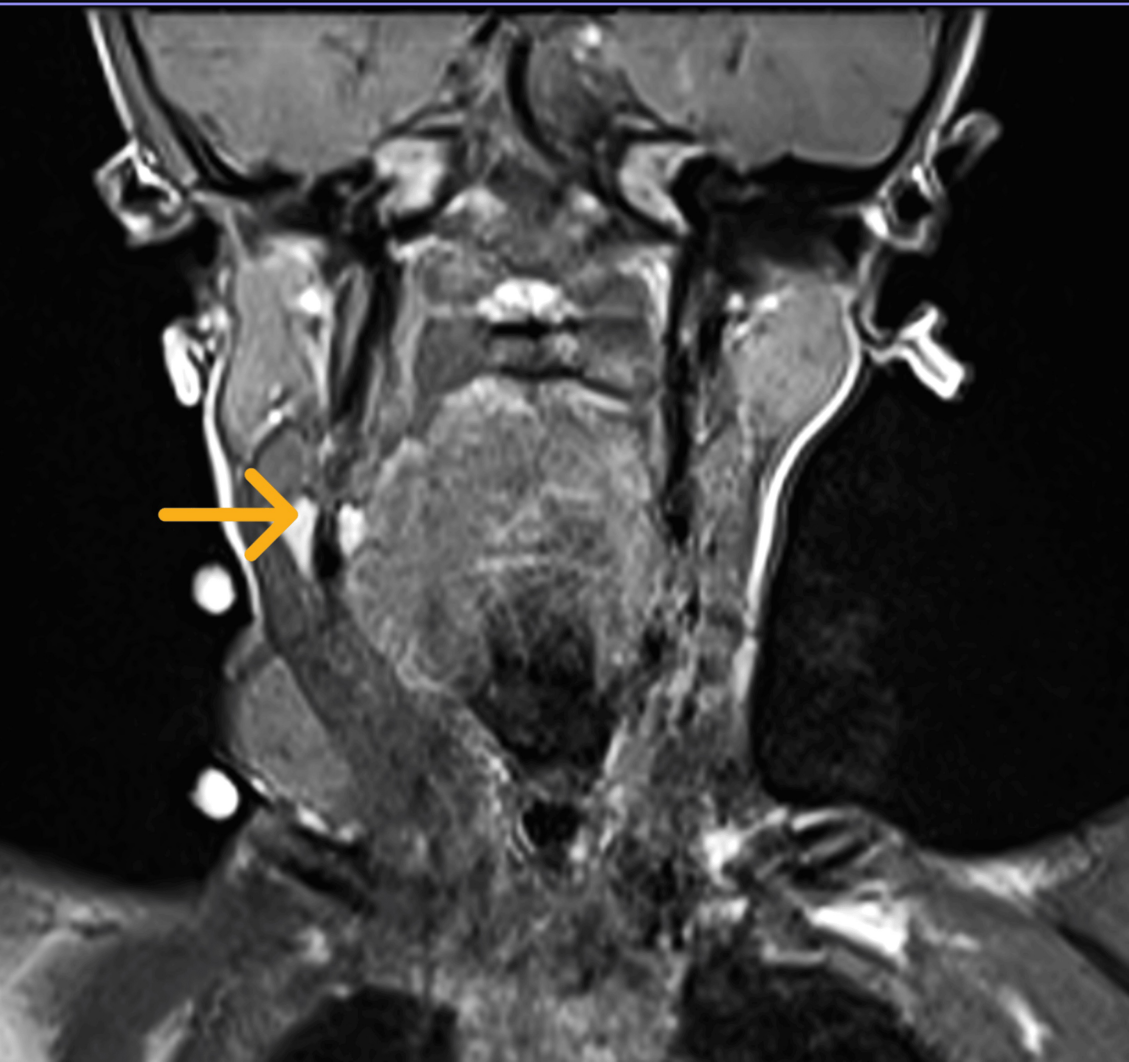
A coronal image of the MRI neck T1-weighted image with a hypointense signal (arrow).
